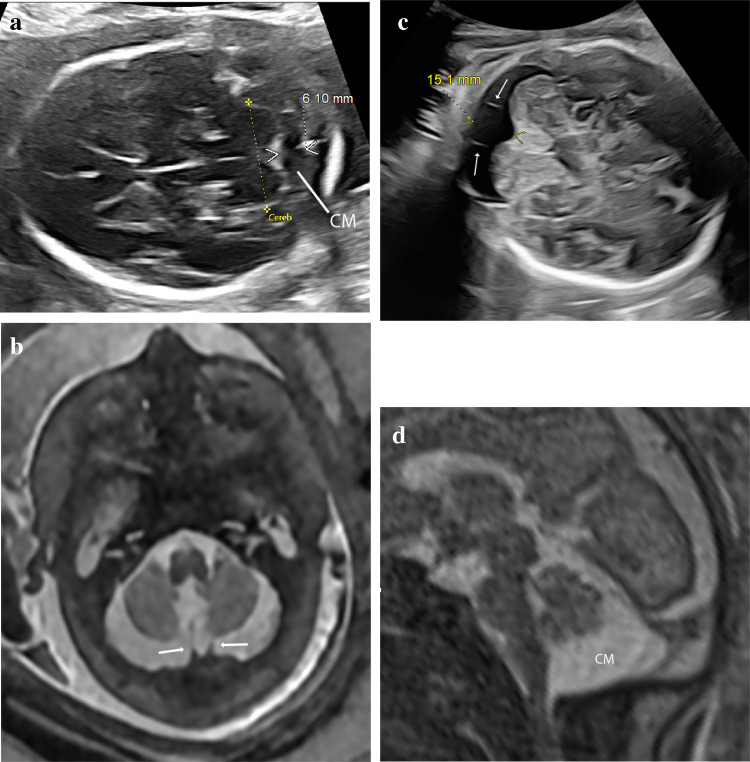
Normal cisterna magna and mega cisterna magna. a Suboccipitobregmatic transverse view of the fetal head at 22 0/7 weeks demonstrates TCD (*between calipers*) and AP measurement of the cisterna magna (*between arrowheads*), normal at 6.1 mm. b Axial T2 single-shot fast-spin echo (SSFSE) through the lower fetal posterior fossa at 24 2/7 weeks shows the typical appearance of the falx cerebelli (*arrows*). c-d Mega cisterna magna in a fetus at 30 0/7 weeks gestation. Transverse ultrasound (c) shows a widened cisterna magna measuring greater than 10 mm in AP dimension. Note linear echogenicities (*arrows*) of the falx cerebelli. Duplication of the falx cerebelli without deviation is supportive of mega cisterna magna. Fetal brain MRI sagittal SSFSE image (d) of the midline posterior fossa at the same gestational age demonstrates abnormal uniform prominence of the cisterna magna (CM). No mass effect or cyst wall was evident, and the parenchyma was normal
